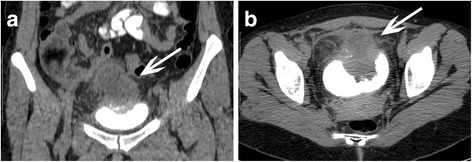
Pelvic CT scan at the time of diagnosis. Tumor mass is demarcated from the contrast-filled bladder (*white arrow*). a Coronal plane. b Transverse plane
